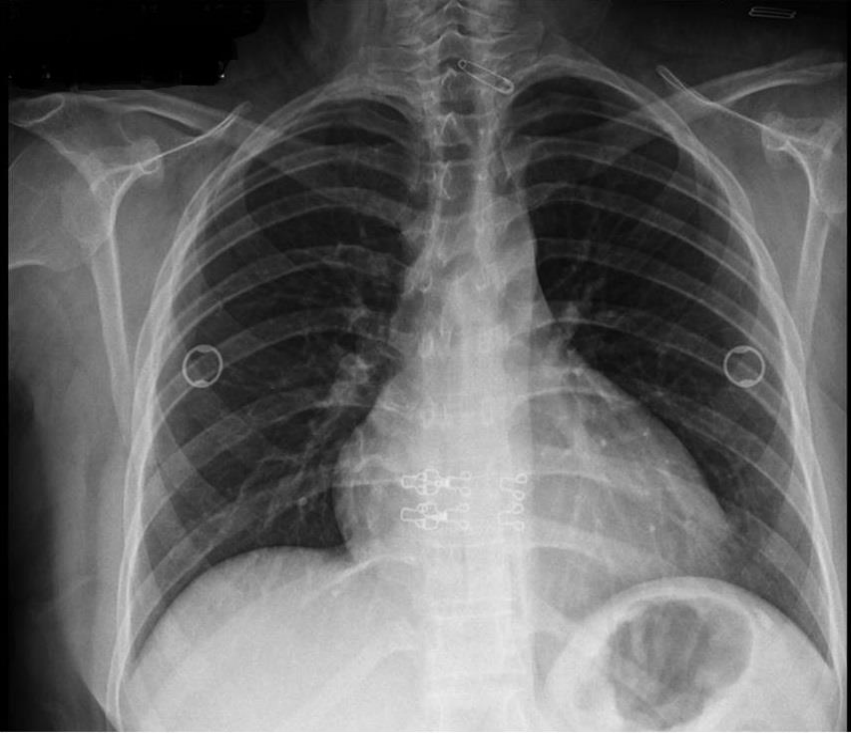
A PA chest radiograph at the second admission demonstrates increased cardiothoracic ratio which suggests cardiomegaly or pericardial effusion
